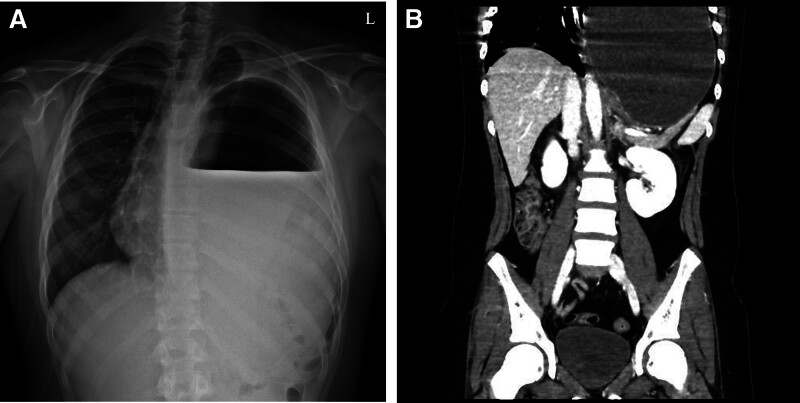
Preoperative imaging in case 7. (A) Chest x-ray shows an opacified left hemithorax with an air-fluid level, mimicking pleural effusion. (B) CT scan reveals a severely distended, herniated stomach within the thoracic cavity. CT = computed tomography.
